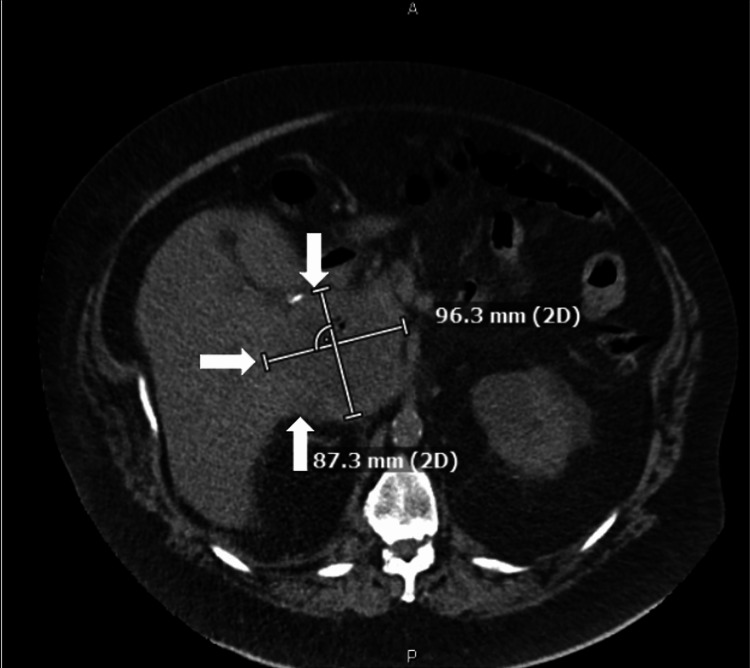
CT of the abdomen/pelvis without contrast revealing a 9.63 x 8.73 cm mass (indicated by arrows) involving the porta hepatis CT: computed tomography
